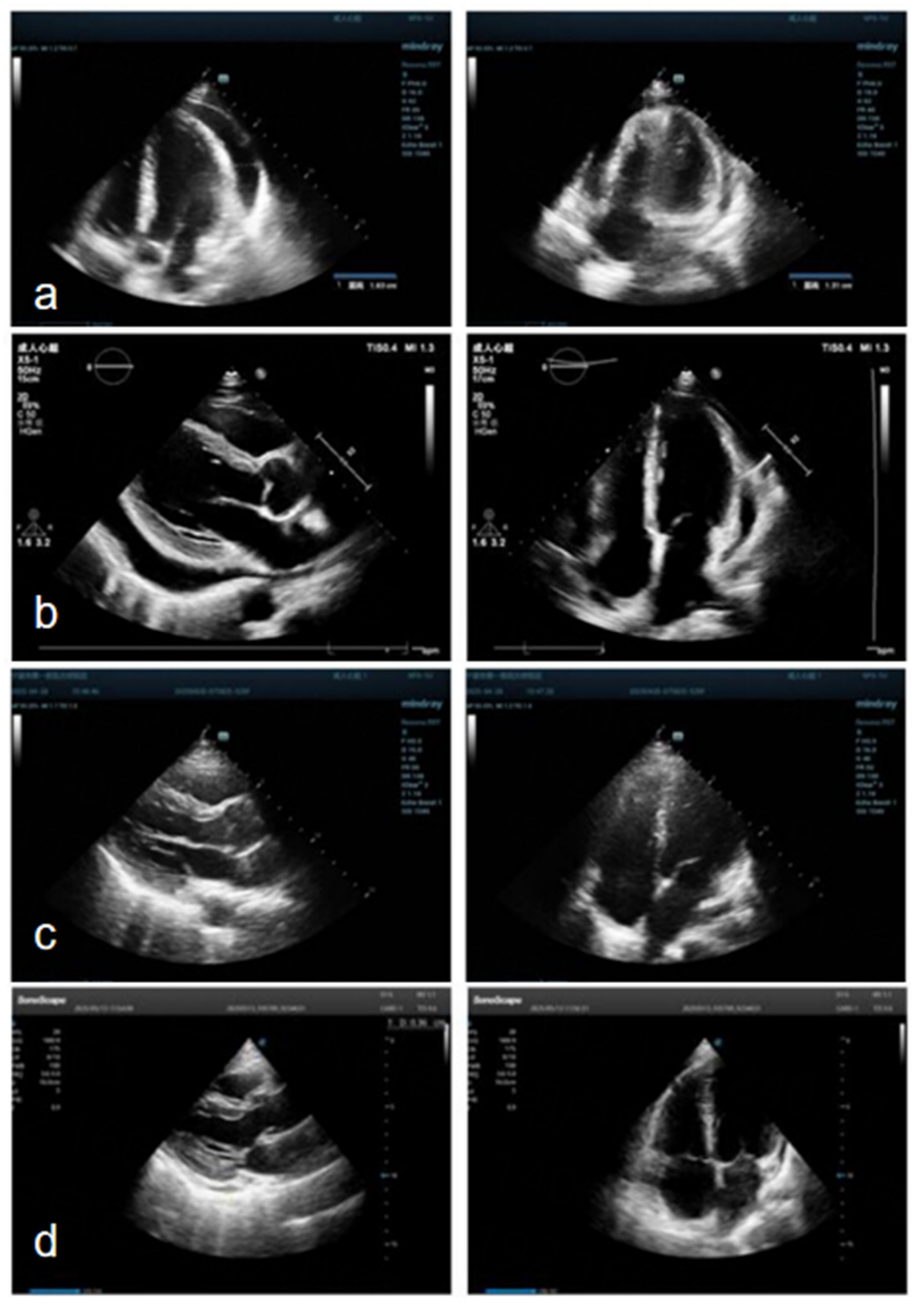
Echocardiography on admission revealed a moderate pericardial effusion along the left ventricular lateral wall, measuring 16 mm at its maximum diameter (a). During hospitalization, repeat echocardiography demonstrated progression to a large posterior left ventricular effusion, measuring 21 mm at its maximum diameter (b). Follow-up echocardiography performed 2 weeks after discharge showed complete resolution of the effusion (c). At 4 months post-discharge, a small residual pericardial effusion measuring 4 mm was observed along the posterior left ventricular wall (d).
